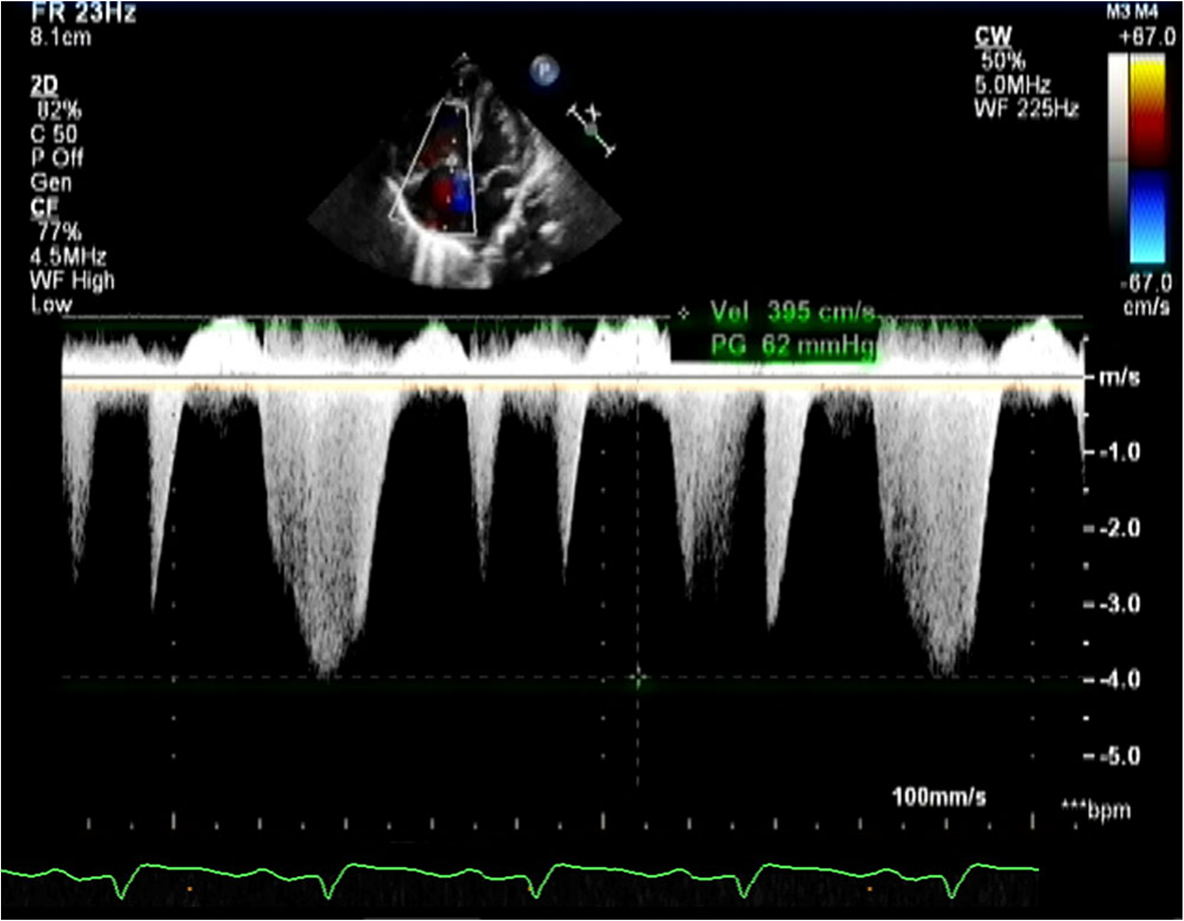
IHH (Infantile hepatic hemangiomas) on abdominal ultrasound, in the left hepatic lobe. CDFI(Color doppler flow imaging) can be detected with abundant blood flow signals
